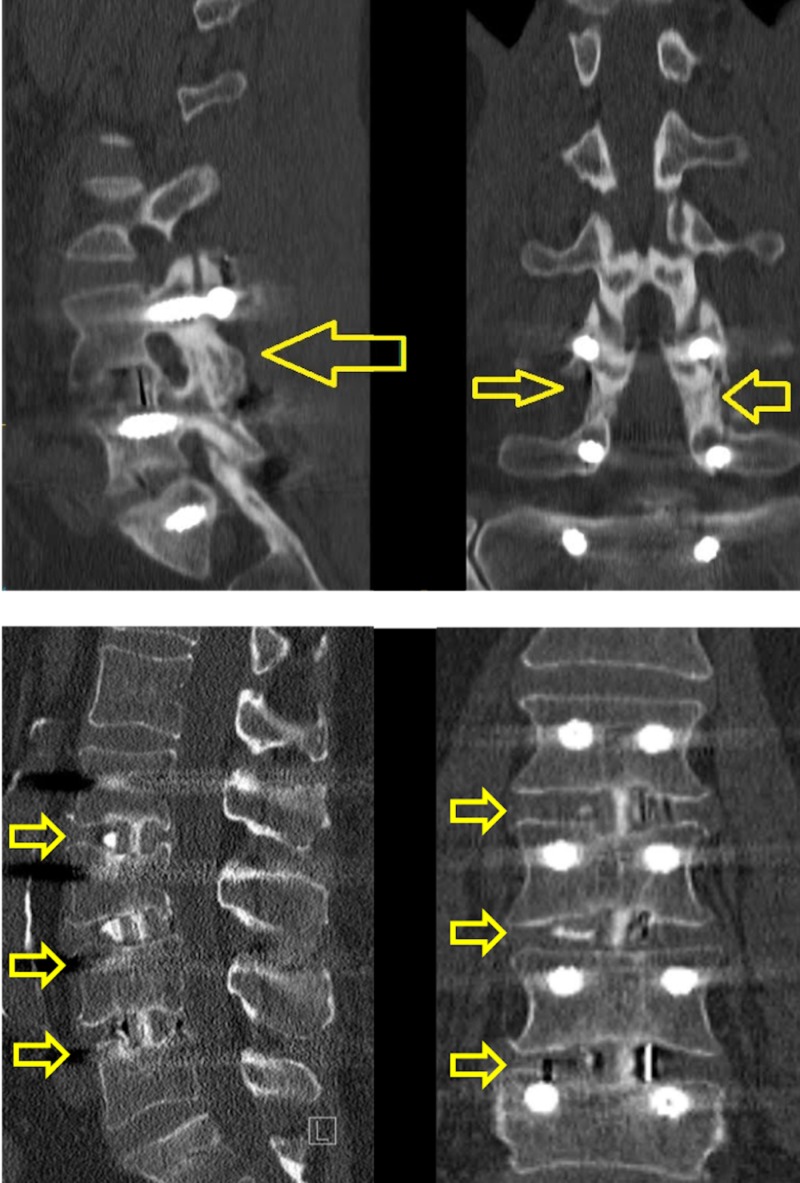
Representative CT scans one year after OLLIF surgery demonstrating (top) posterolateral fusion, (bottom) interbody fusion. Arrows indicate levels where fusion has occurred. CT: Computed tomography; OLLIF: Oblique lateral lumbar interbody fusion
